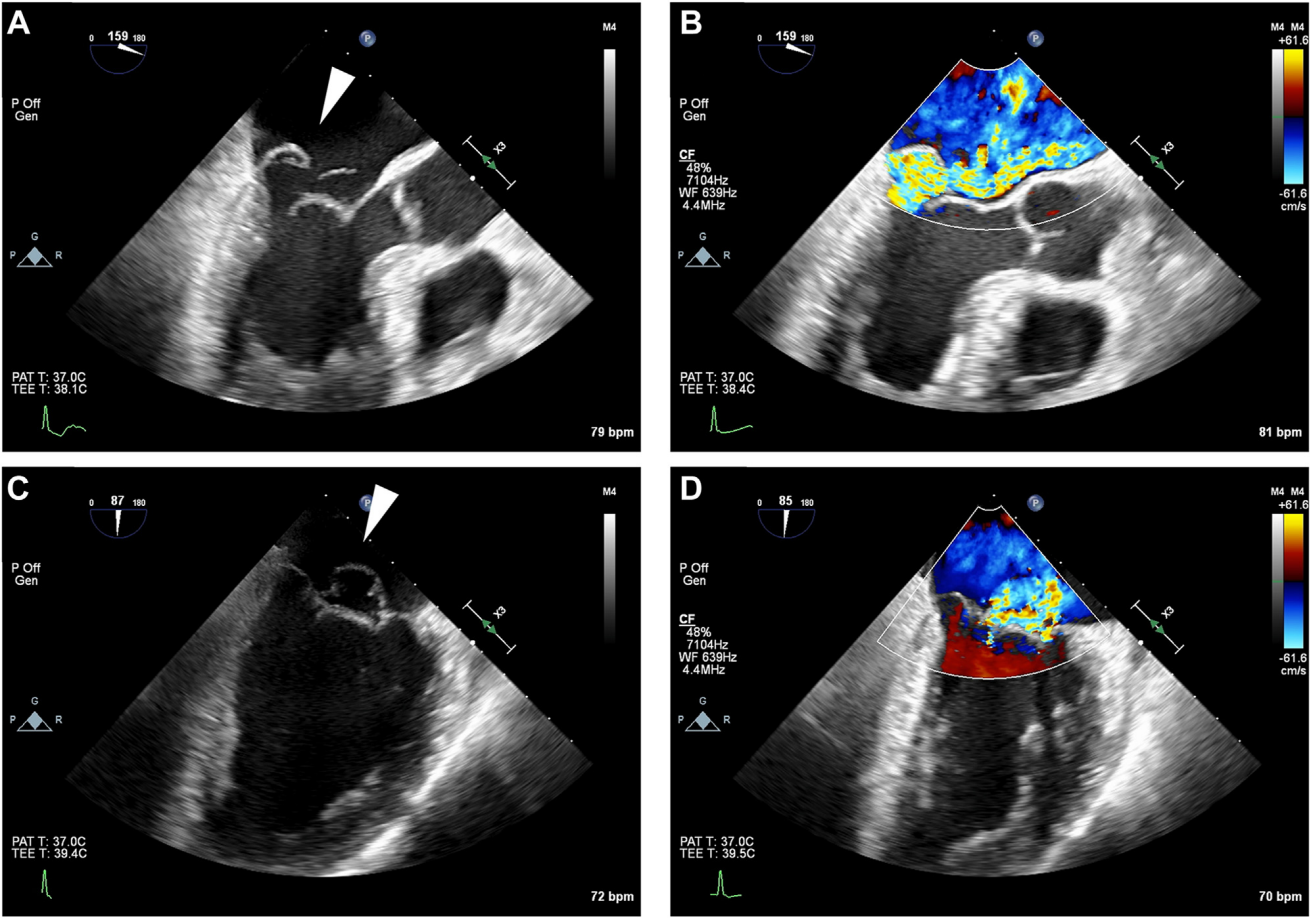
Preoperative transesophageal echocardiography findings. (A, B) Midesophageal aortic valve long-axis view. (C, D) Midesophageal mitral commissural view. (B, D) Echocardiography color Doppler images. The arrowheads in (A) and (C) indicate P2 prolapse with chordal rupture, and severe mitral regurgitation is assessed by color Doppler in (B) and (D).
